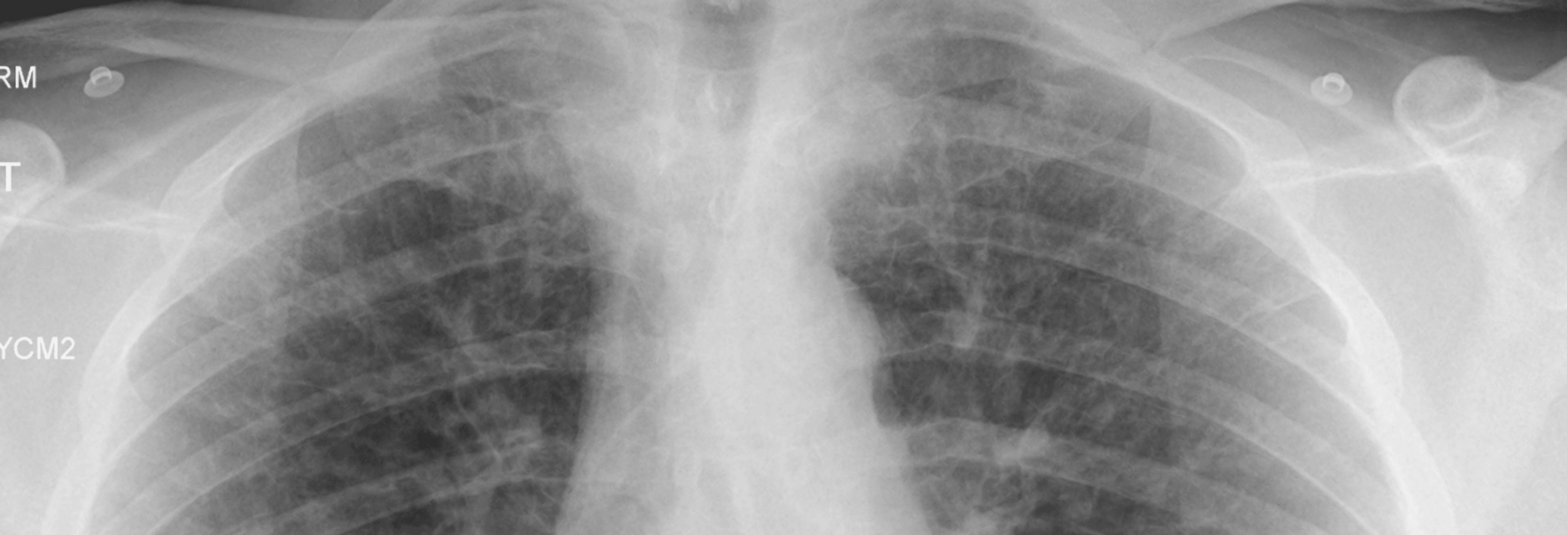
Post-angiogram CXR showing the apical zones only Accentuated lung markings are seen in the upper zones. CXR, chest X-ray
